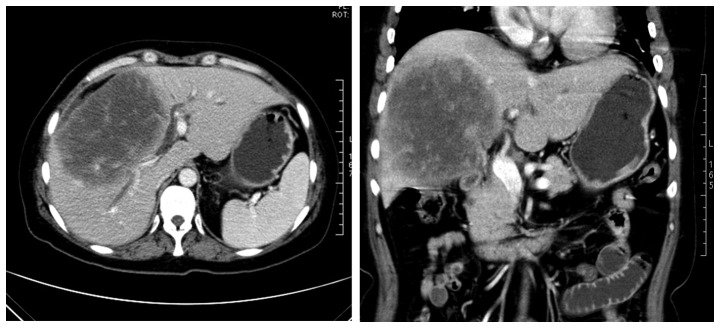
CT images of a patient with large liver tumor. The right hepatic bile duct was invaded and the left bile duct was slightly expanded due to tumor compression.
